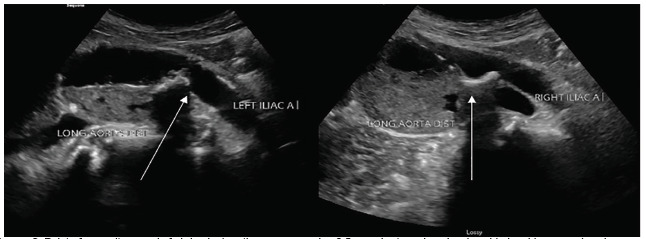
Point-of-care ultrasound of abdominal aortic aneurysm using 3.5 megahertz probe, showing side-by-side comparison in transverse view of the aorta, at the level of bifurcation of the left and right iliac arteries as labeled. Dislodged clot can be seen at the level of bifurcation shown above (arrows).
